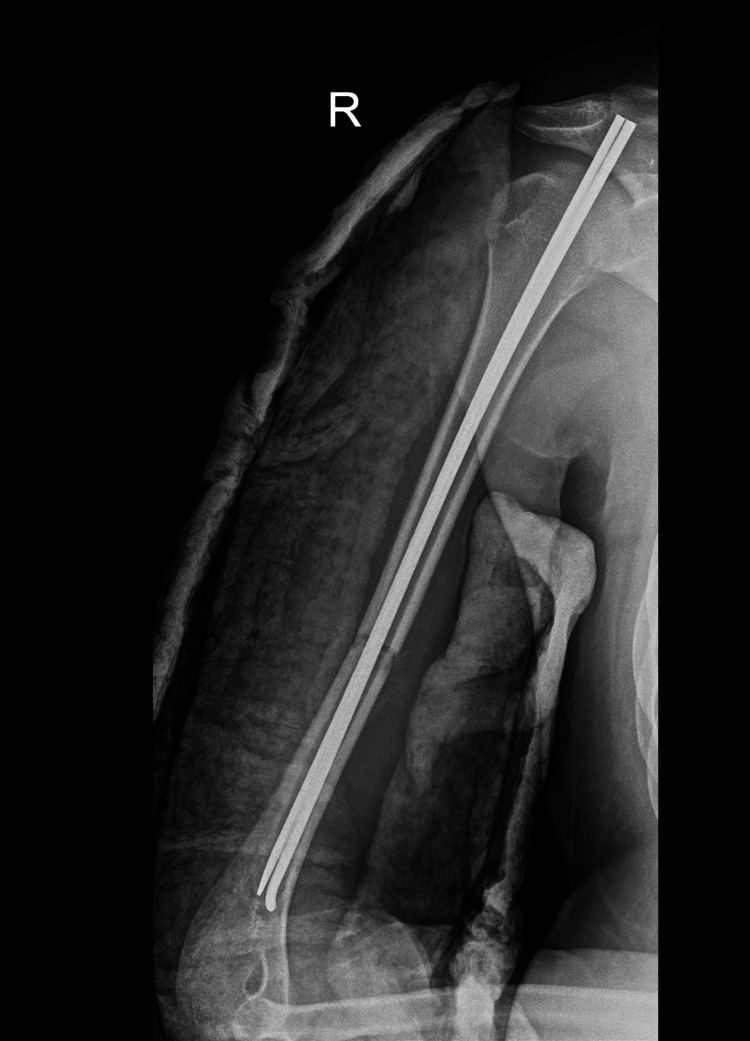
X-ray four weeks post-surgery
